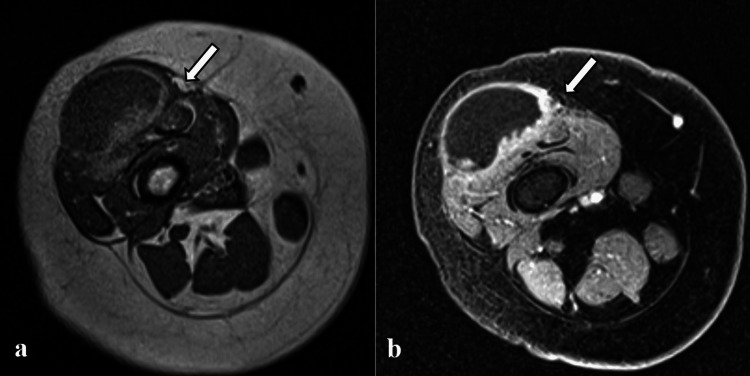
T1 MRI of the thigh, axial view, before (a) and after (b) contrast. Before contrast administration (a), the cystic mass is visualized over the anterolateral thigh, with a solid component evident along its posterior aspect (arrow). After contrast administration (b), there is an enhancement of the capsular walls and the solid component (arrow).
